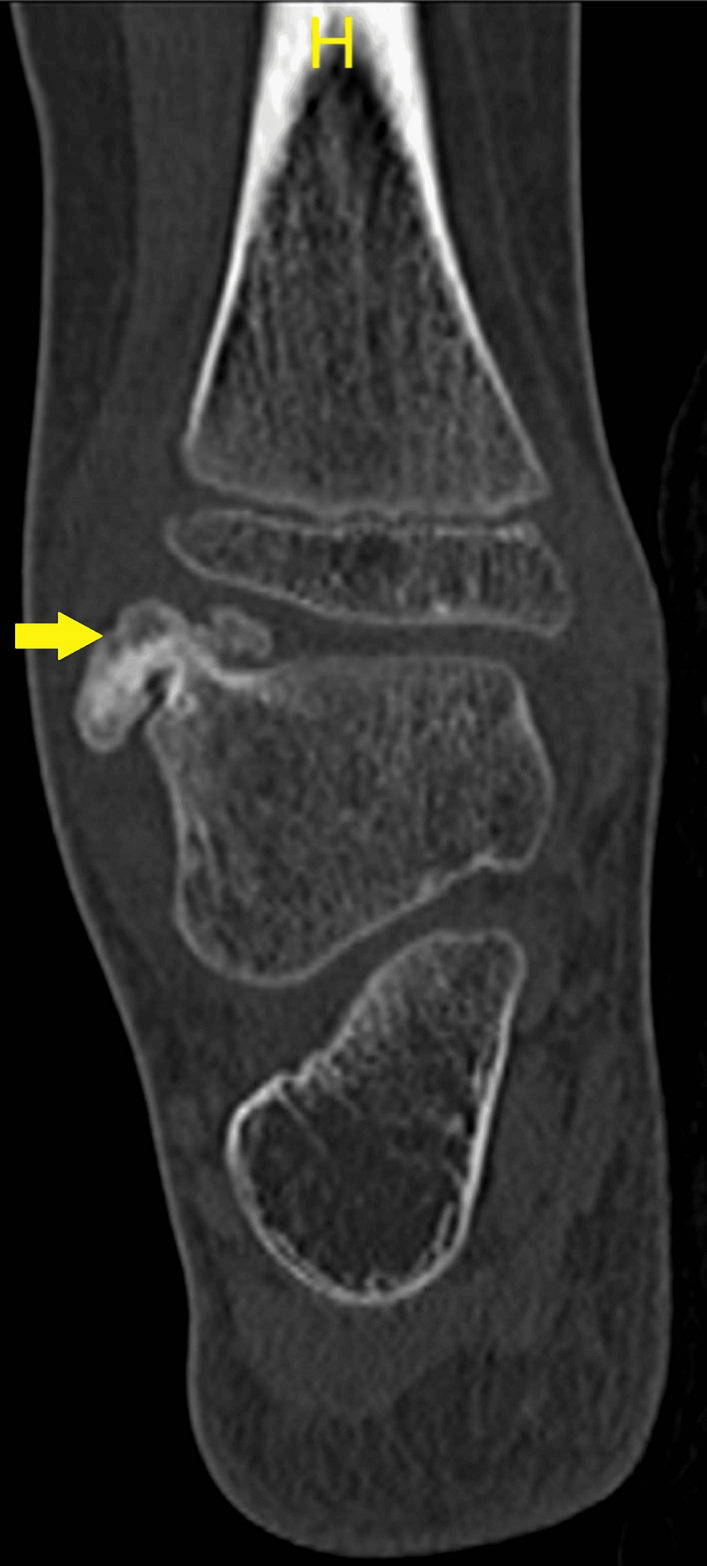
CT coronal view: arrows demonstrate the osteochondromas from the posterior aspect of the talus and in the interosseous region
